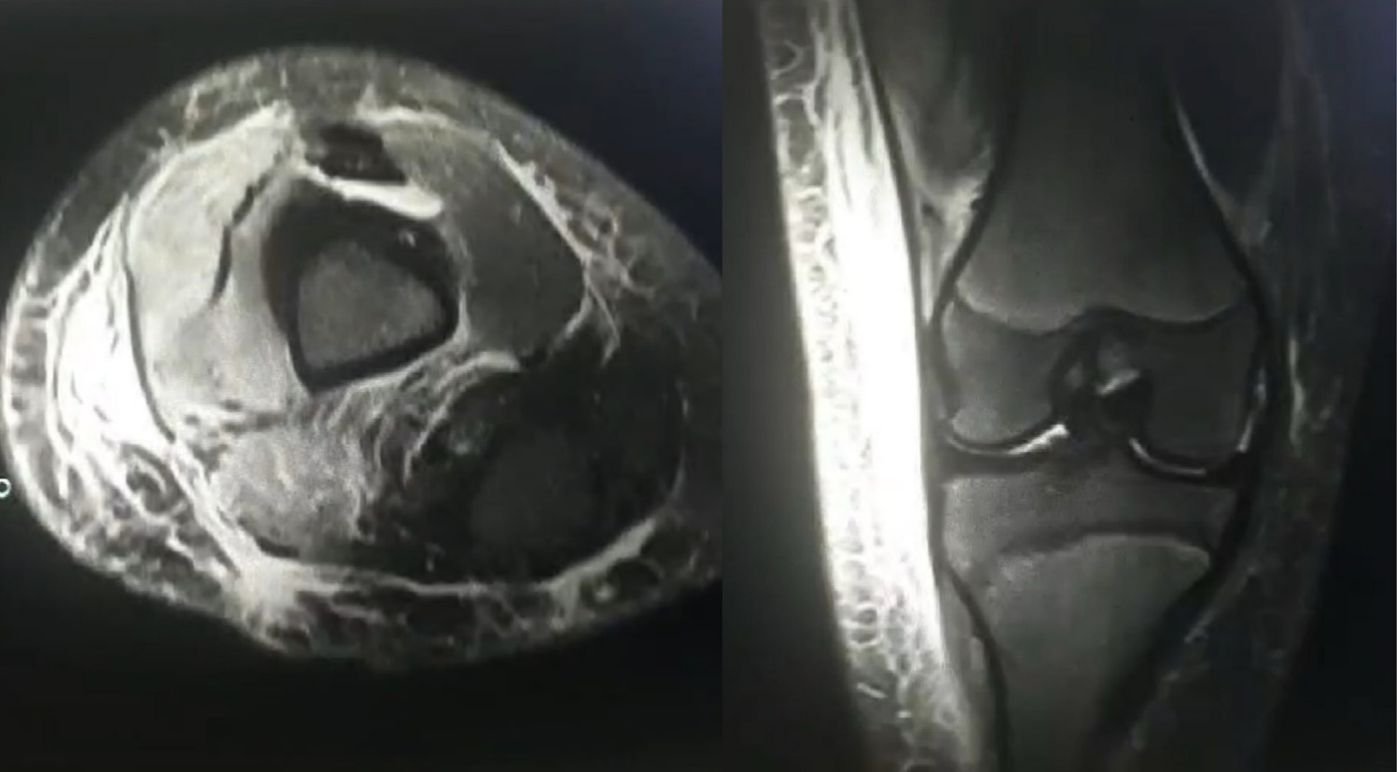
Axial and coronal MRI sections showing significant subcutaneous and muscle edema indicating myositis with no intramuscular collection.
